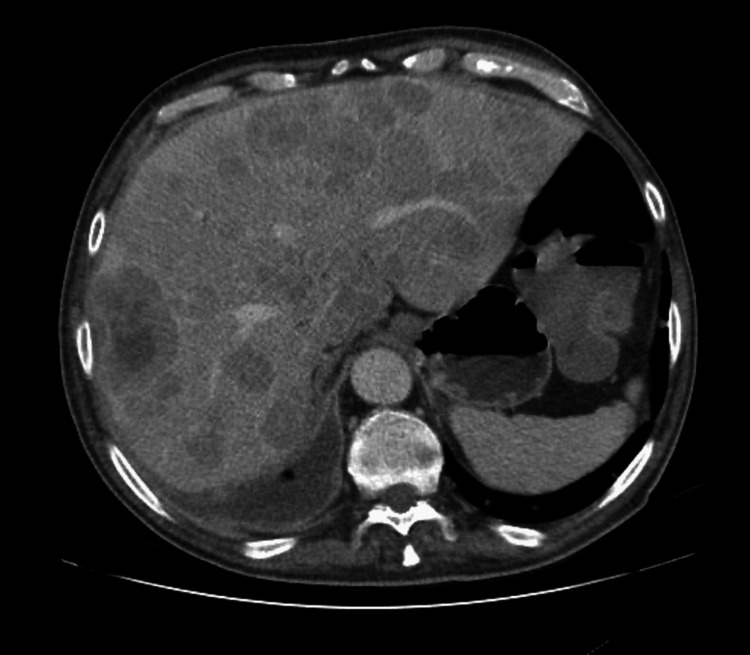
Follow-up abdominal CT after three cycles on docetaxel and carboplatin (Case 1) Progression of hepatic disease.
